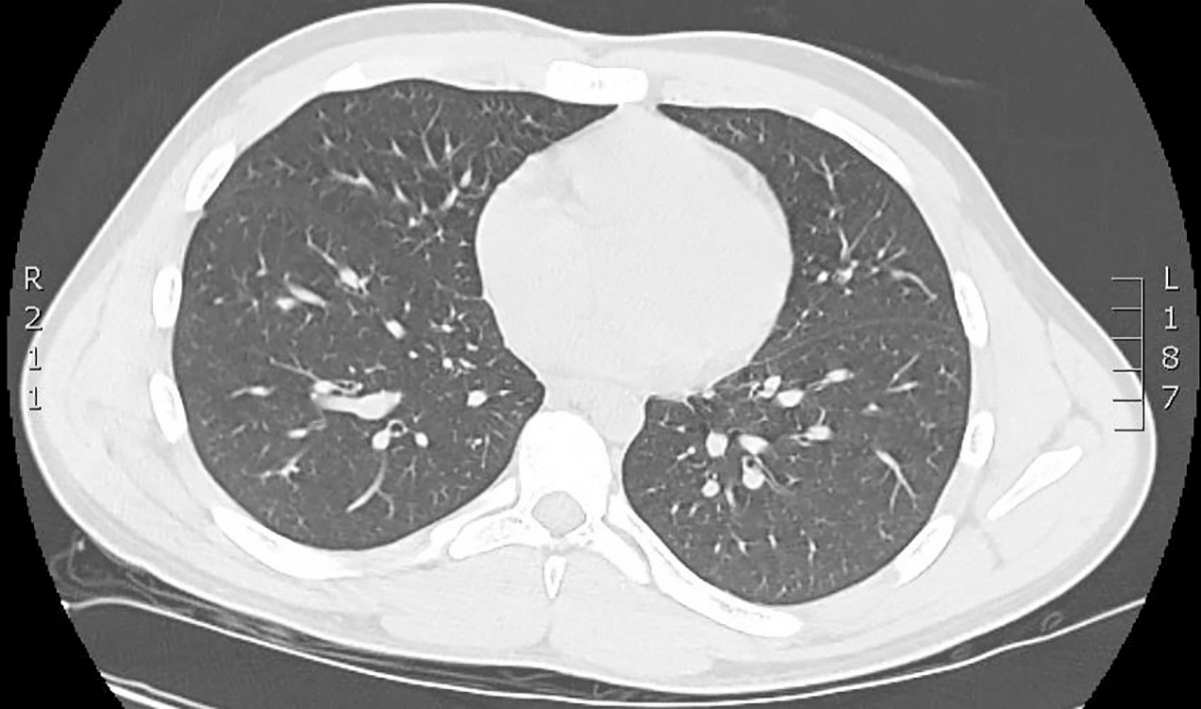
CT scan at admission was normal. CT, computed tomography.
